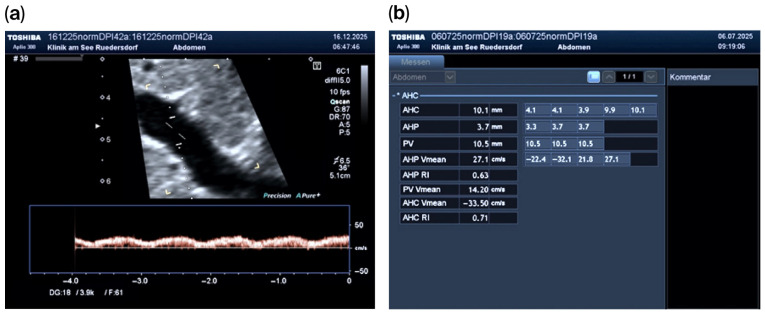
Doppler sonographic approach to the portal vein. (a) Doppler spectral waveform of the portal vein. (b) Documentation of measurement results using the standardized ultrasound preset. Note. Measurements were performed using an intercostal approach. Values of 9.9 mm and 10.1 mm represent portal vein diameter measurements.
